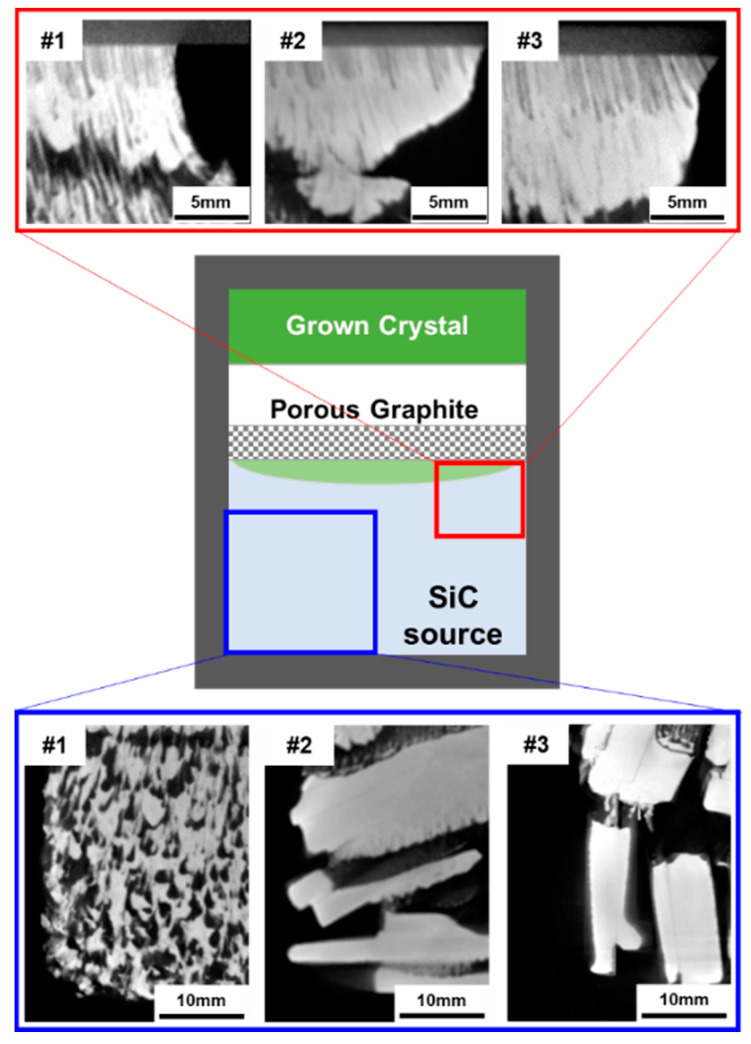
X-ray CT images of remaining CVD-SiC block sources after PVT growth in the source regions.
